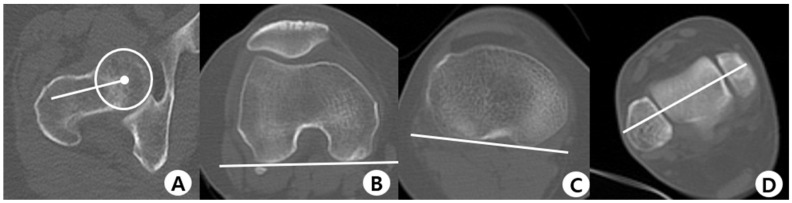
Axial computed tomography. (A) Femoral neck axis; (B) The line adapted to the posterior contour of the medial and lateral femoral condyles; (C) The line adapted to the posterior contour of the proximal tibial head; (D) The bimalleolar axis.
